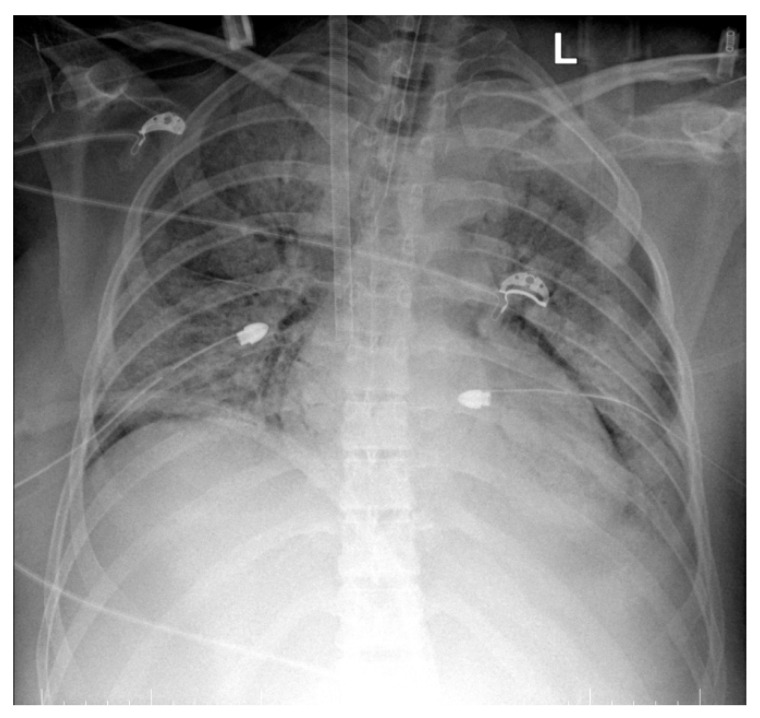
Thoracic X-Rays showing an improvement in the radiological aspect of the lungs after ECMO support was instituted (L-left).
